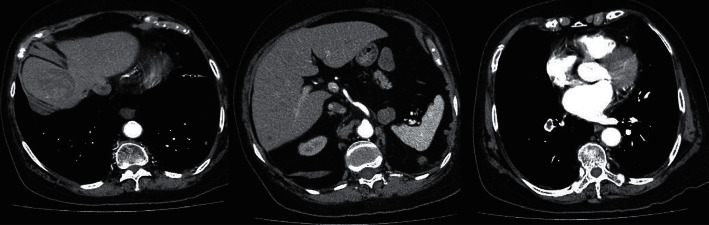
CT abdomen of Patient B on 30 December 2016. (a) Presence of liver metastasis. (b) Nodule at left adrenal bed and peritoneal nodule posterior to spleen. (c) Right descending pulmonary artery embolism.
